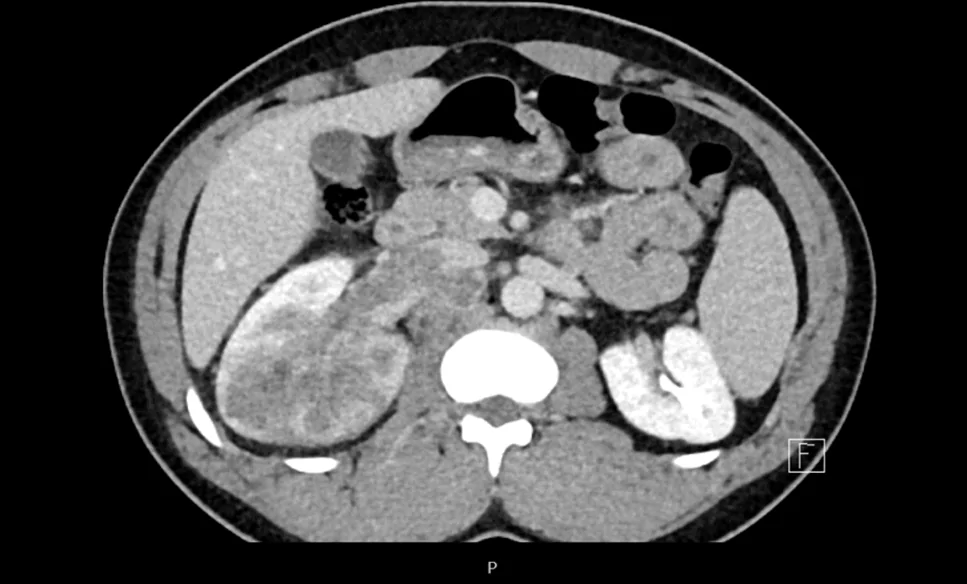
29-year-old Black female with sickle cell trait presenting with haematuria. Axial contrast-enhanced venous-phase CT scan shows an infiltrative tumour replacing the right kidney, extending into the renal vein and inferior vena cava, with retroperitoneal nodal disease. There were also metastases to the lungs, liver, bone, and supradiaphragmatic lymph nodes (not shown)
